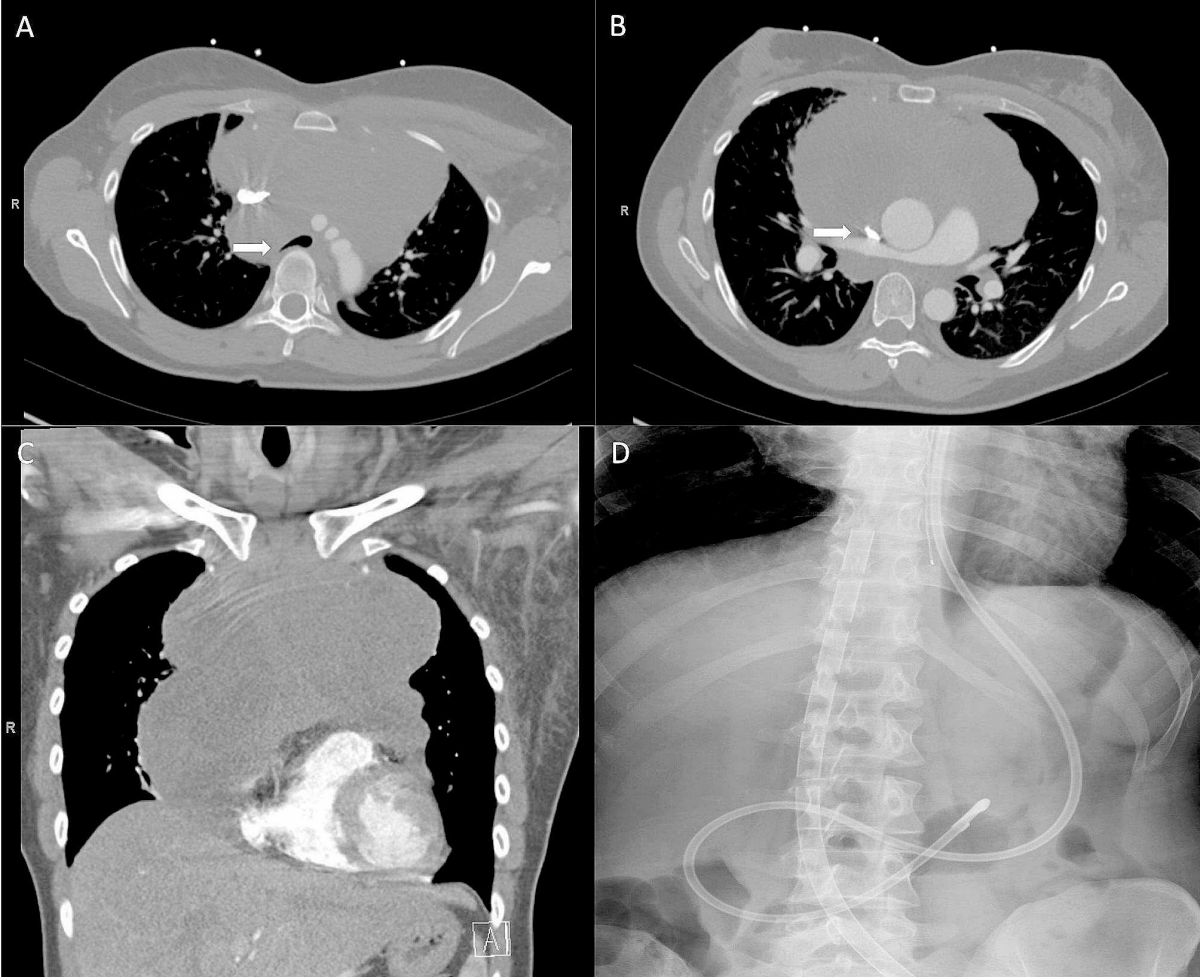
Pretreatment chest computed tomography (A) a mediastinal mass compressing the distal trachea against the vertebral column (white arrow). (B) Stenosis of the superior vena cava compressed by a mediastinal mass (white arrow). (C) a large heterogeneous 12 × 8 × 14 cm soft tissue mass within the anterior mediastinum (D) Xray abdomen after VA ECMO cannulation
